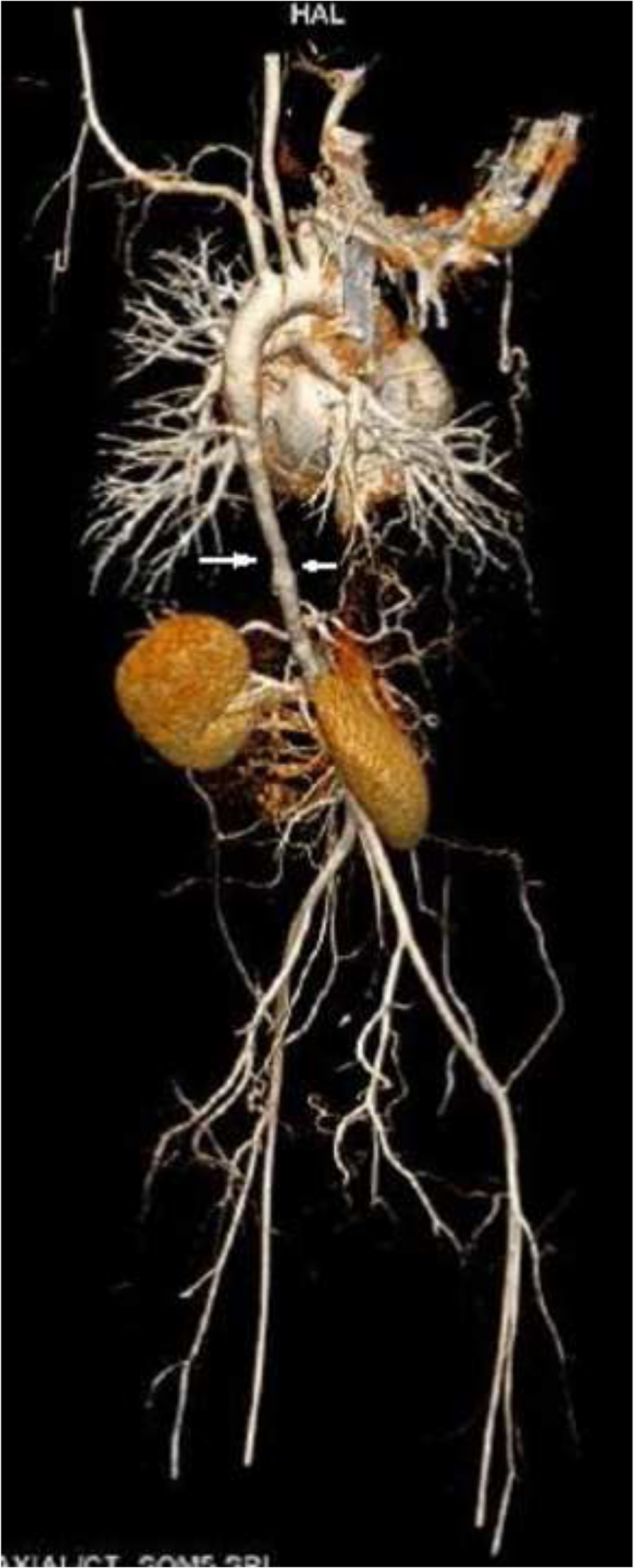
Three-dimensional computed tomography scan of the thoracic and abdominal aorta showing irregular narrowing with variable degrees of stenosis (*arrows*), tapering and corrugated appearance
